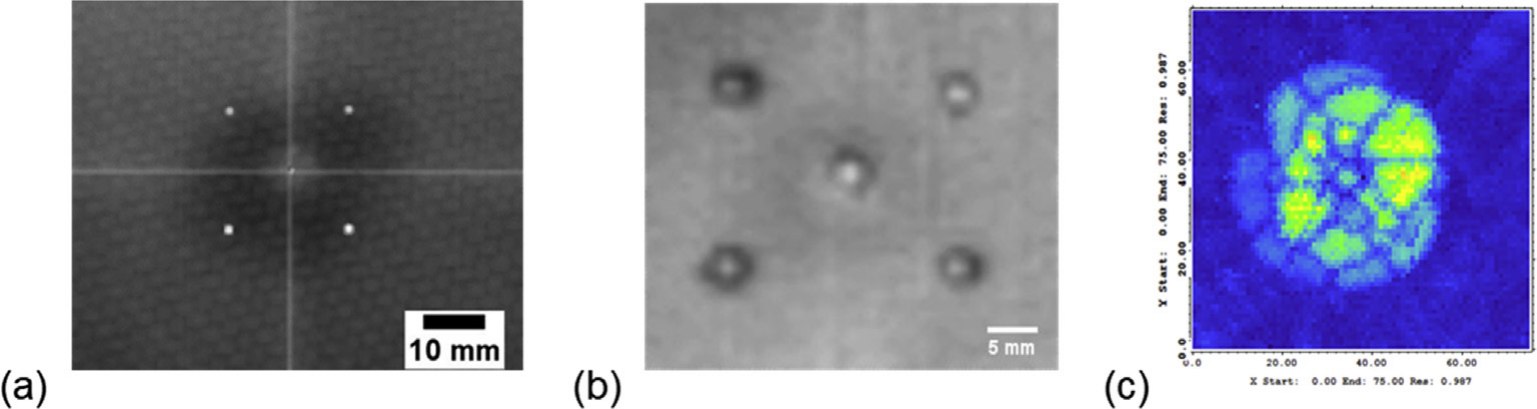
Images of the BVID CFRP laminates acquired by ultrasonic C-scan and Infrared thermography. (a) Thermogram of BVID in a CFRP laminate. (b) Magnified view of the thermogram in panel a, revealing the damage around the holes. (c) C-scan image of BVID in CFRP laminate.
